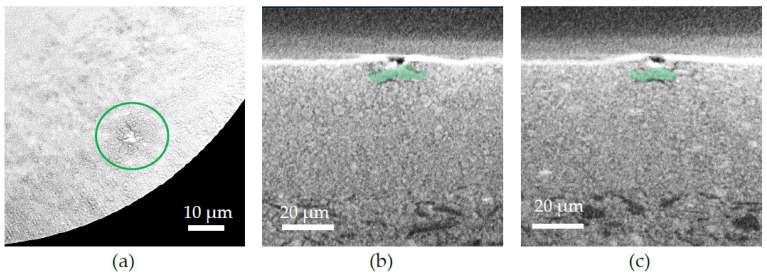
X-ray tomography images of a defect in the upper layers of the raw sample: (a) image from the top (*Y*-axis) of the defect in the electrolyte; (b) cross-section where the defect is along the *X*-axis with a NiO grain (in green) in the AFL; (c) cross-section where the defect is along the *Z*-axis with a NiO grain (in green) in the AFL.
